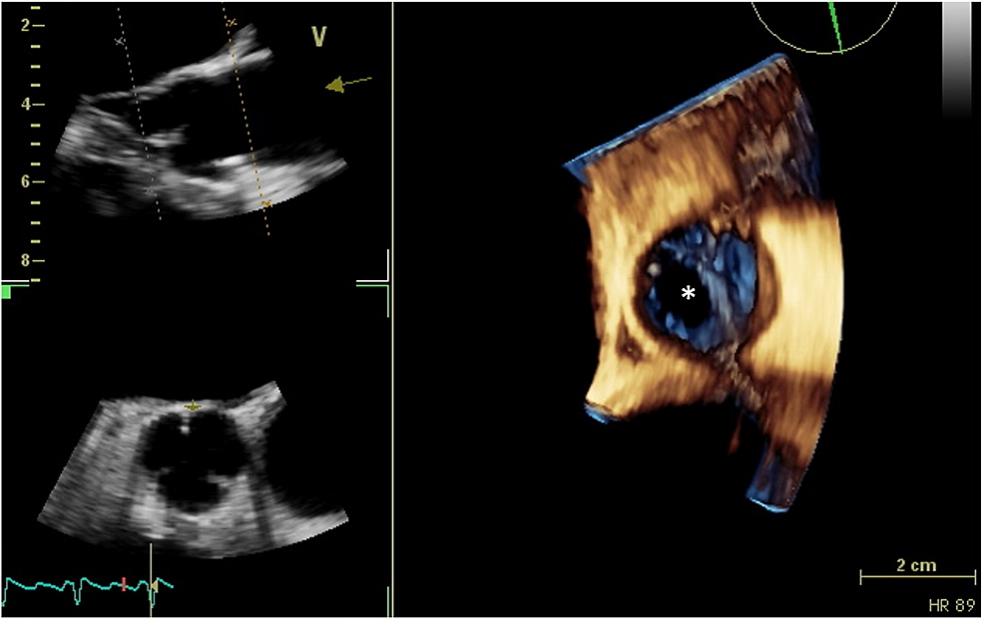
Vegetations of the aortic valve cusps with non-coronary cusp perforation (asterisk) in transesophageal echocardiogram
